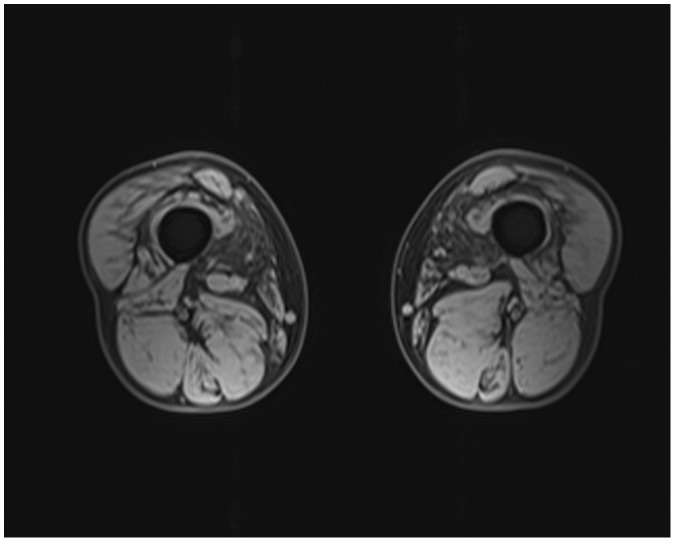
Muscle MRI imaging of the index case showing slight atrophy in the lateral and medial muscles of the thigh.
